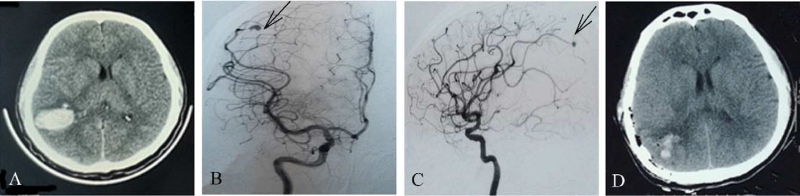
Patient's imaging data during the first ICH. (A) The head CT showed ICH in the right parietal lobe. (B, C) The head DSA (B: anteroposterior view; C: lateral view; black arrow) showed an aneurysm of the distal right middle cerebral artery (M4 segment). (D) The head CT showed that the hematoma was cleared after the first operation (electrocoagulation molding of aneurysm and wrapping). CT = computer tomography, DSA = digital subtraction angiography, ICH = intracerebral hemorrhage.
